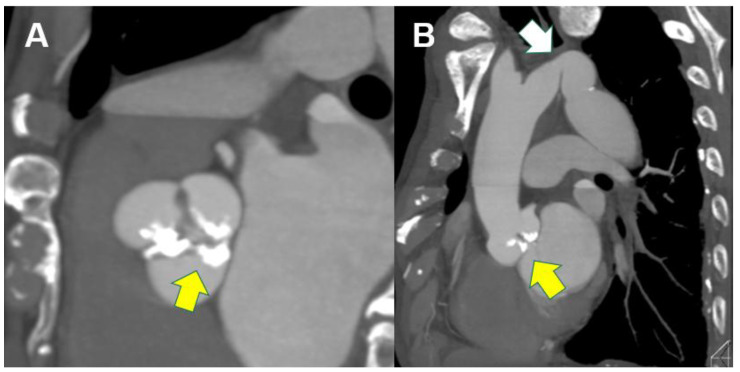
Thoracic aortic coarctation in a case with severe aortic valve stenosis. These were maximum-intensity projection images of computed tomography in a case with severe aortic valve stenosis and aortic coarctation. Panel (A) shows severe aortic valve calcifications on a short axial image of the aortic valve. Panel (B) shows coarctation of the aortic arch and severe aortic valve calcifications. Yellow arrows indicate a highly calcified aortic valve. A white arrow indicates aortic coarctation.
